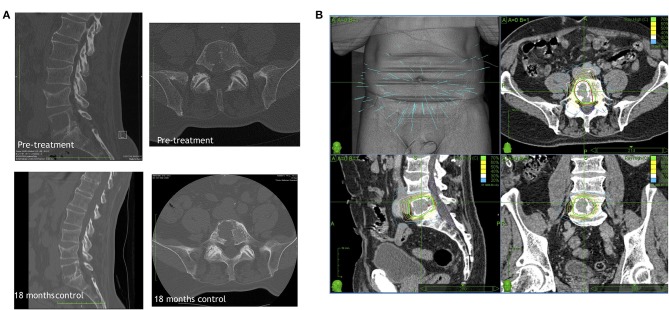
A representative primary Cyberknife treatment case showing (A) the CT scan of the lesion before and 18 months after the treatment visualizing the stable lesion and (B) the Cyberknife therapy planning.
